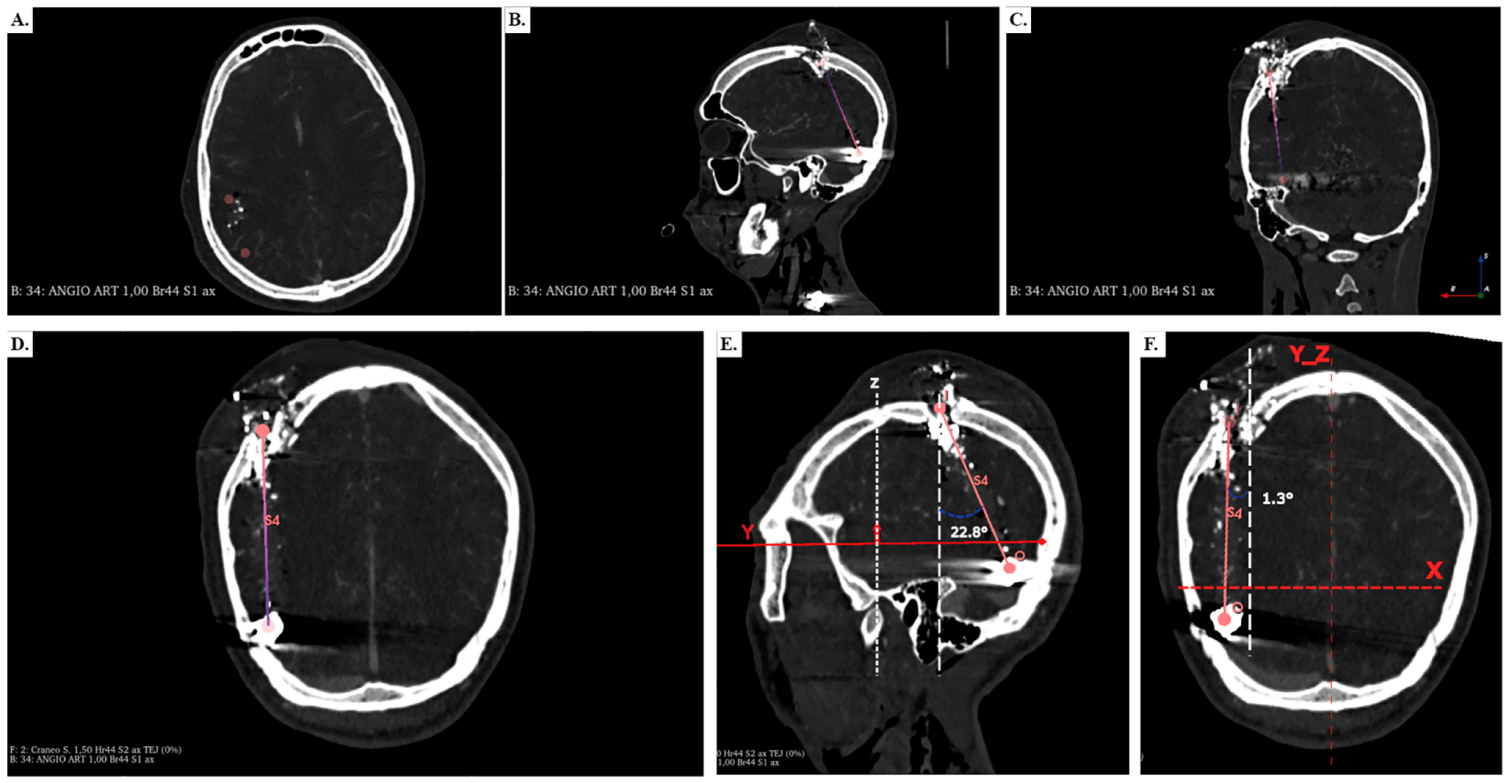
Comparison between the axial, sagittal and coronal views (classical views), regarding the para-axial, para-sagittal and para-coronal views of patient S4, a survivor, for determining resultant pathways in trajectories. a. Axial view. b. Sagittal view. c. Coronal view. d. Para-axial MPR showing trajectory 67.07° with respect P_Axi_RA (RA Axial plane), seems like the para-coronal plane. E. Para-sagittal CT MPR showing trajectory 22.8° toward the occipital bone, demonstrating the para-coronal orientation of the wound path. F. Para-coronal CT MPR showing trajectory 1.3° toward the patients Right, demonstrating the para-sagittal orientation of the wound path
